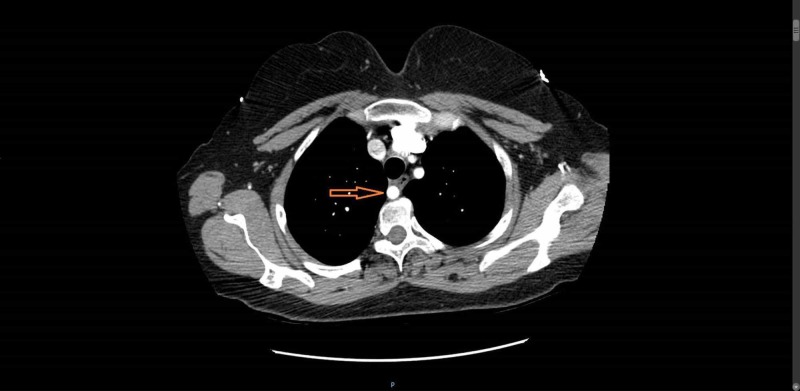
Chest CT scan showing retro-esophageal aberrant right subclavian artery.
